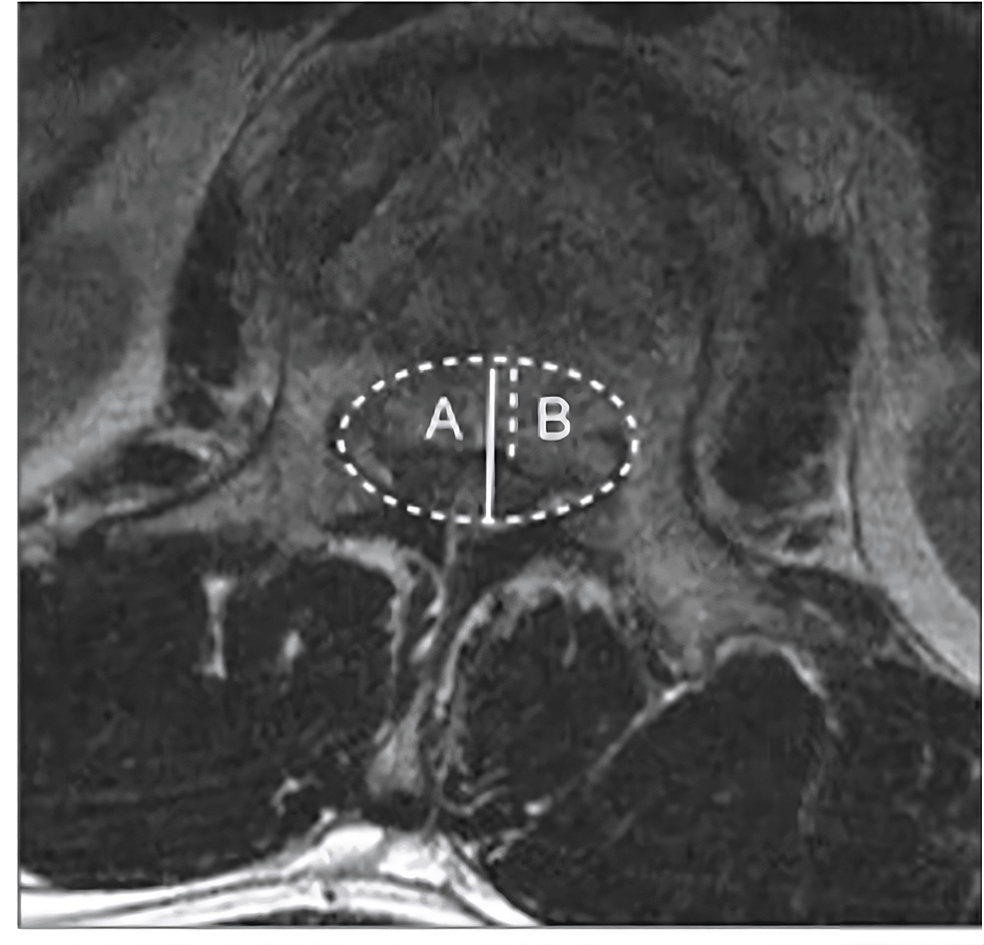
The neural canal encroachment ratio is measured by the ratio of retropulsion bony fragment anteroposterior diameter and central canal anteroposterior diameter in the CT axial plane.
